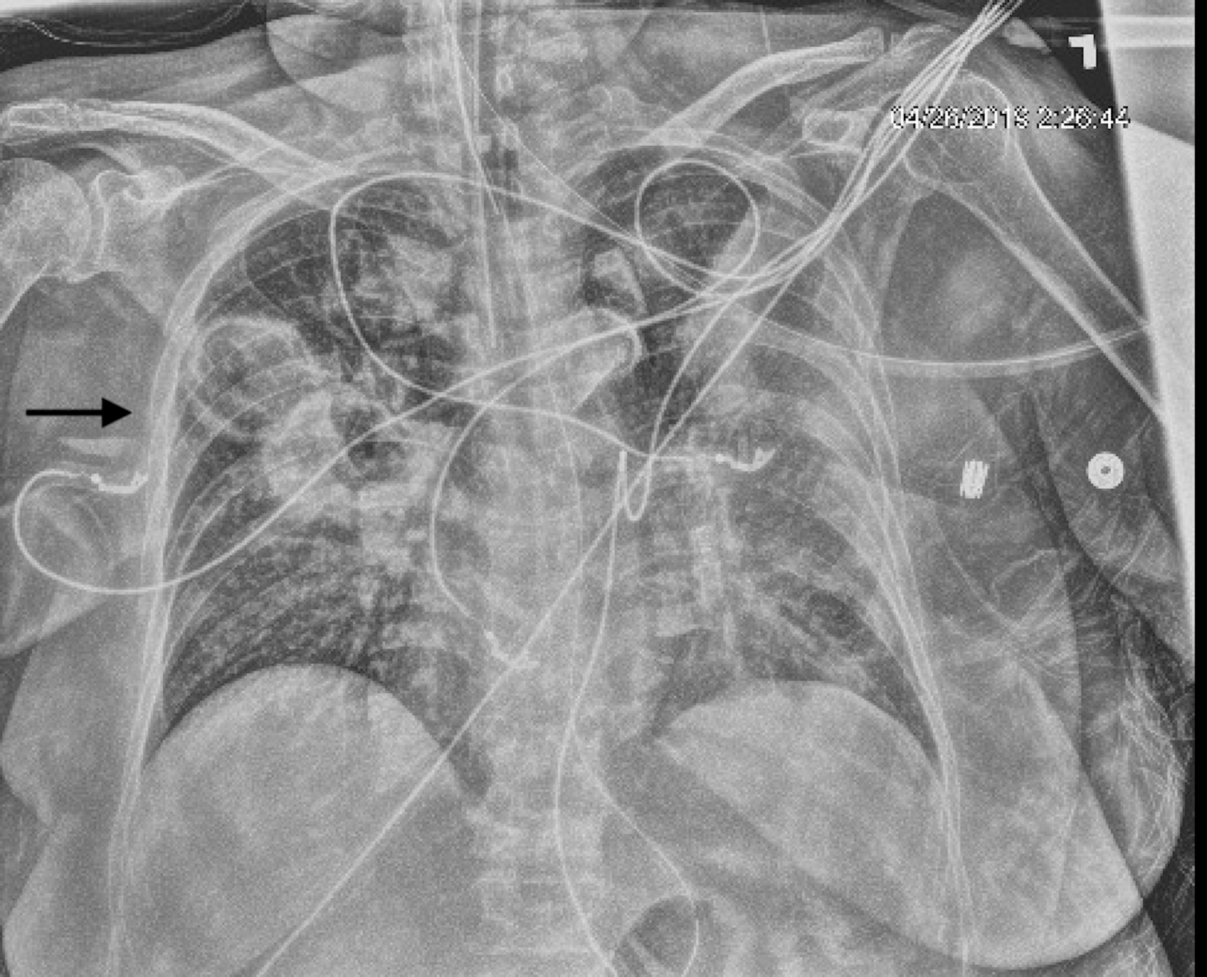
Chest X-ray showing right upper lobe cavitary lesion with a surrounding thick wall
